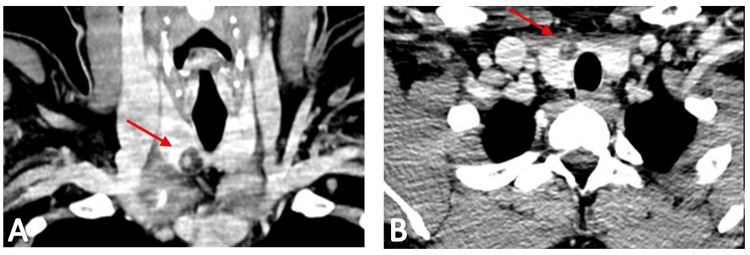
Coronal (A) and axial (B) views of CT scan showing a thyroid gland nodule Head and neck CT scan showing a cystic nodule (arrows) within the right thyroid lobe. CT: Computed tomography
